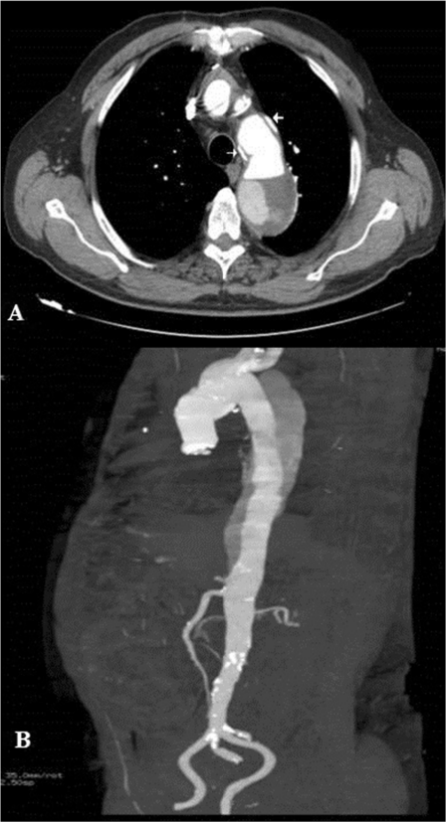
a The contrast enhanced angio-CT at one week after surgery demonstrating the initial segment of the “extroverted cuff”. b The contrast enhanced angio-CT at two years demonstrating a normally functioning elephant trunk. Legend: The arrows demonstrate the “extroverted cuff”
